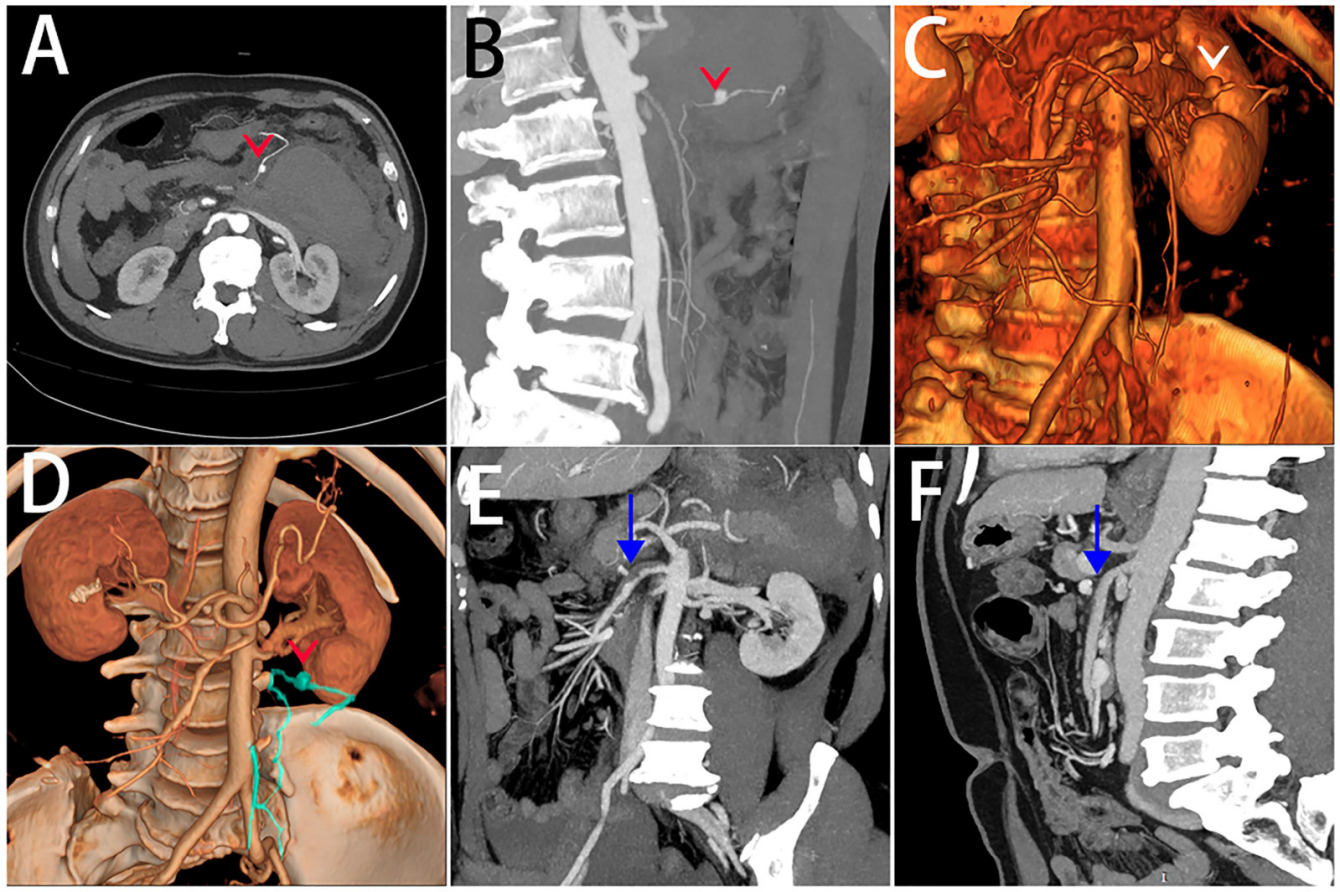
Images in a 57-year-old man with an inferior mesenteric artery aneurysm. (A–D) CT shows abdominal hematoma in the left upper quadrant, and cinematic renderings from CT angiography show a small aneurysm of the inferior mesenteric artery (v). Intestinal artery CTA shows moderate stenosis of the proximal superior mesenteric artery (E↓) and no significant stenosis of the superior mesenteric artery (F↓).
